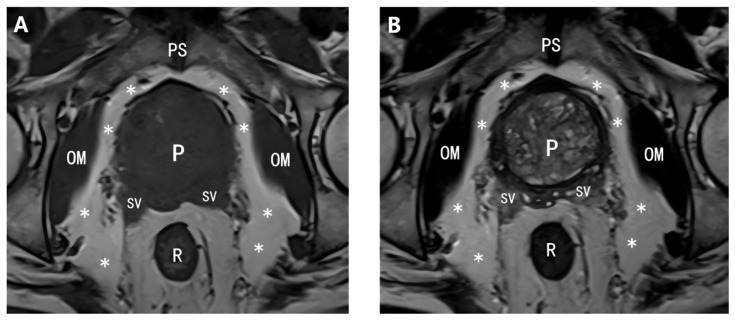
Prostate MR images. (A) Axial T1-weighted MR image of the prostate. (B) Axial T2-weighted MR image of the prostate. The asterisk (*) indicates periprostatic adipose tissue (PPAT). Abbreviations: PS: Pubic symphysis; OM: Obturator internus muscle; P: Prostate; SV: Seminal vesicle; R: Rectum.
